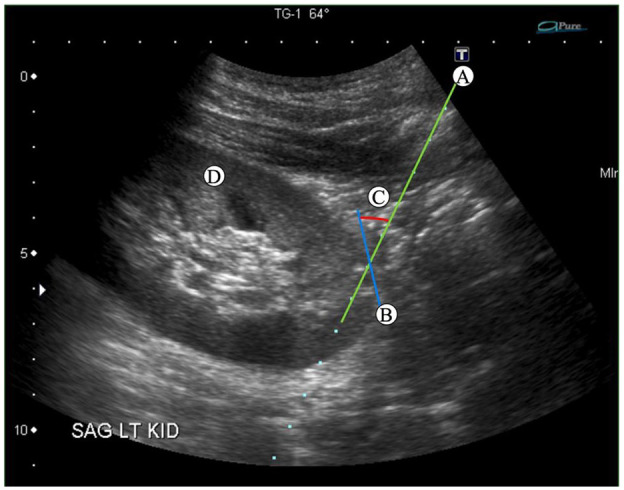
Ultrasound image taken at the time of biopsy. (A) Biopsy needle, (B) tangential line, (C) angle of attack (AOA), and direction of needle in relation to the medulla (D) kidney.
